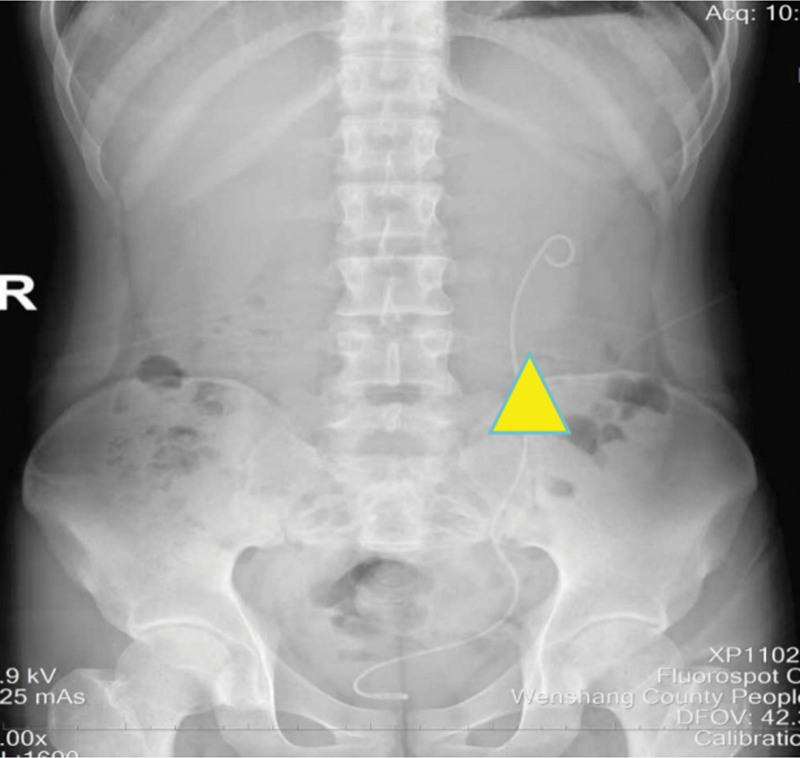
Abdominal radiograph. The placement of the ureteral stent tube is normal (indicated by yellow triangle).
